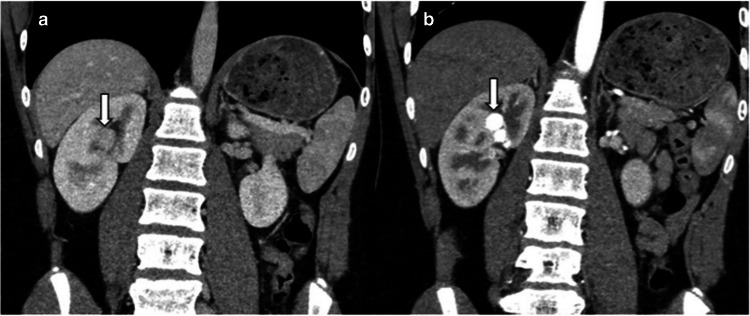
Renal arteriovenous malformation. A 16-year-old boy was admitted to the hospital with hematuria. a Coronal venous phase contrast-enhanced computed tomography (CT) image shows a nodular isodense lesion (*arrow*) in the right renal sinus, mimicking a mass. b Coronal arterial phase contrast-enhanced CT image demonstrates tortuous vascular structures in the right renal sinus with attenuation similar to the aorta, consistent with an arteriovenous malformation (*arrow*). The lesion was treated via embolization in the Department of Interventional Radiology
